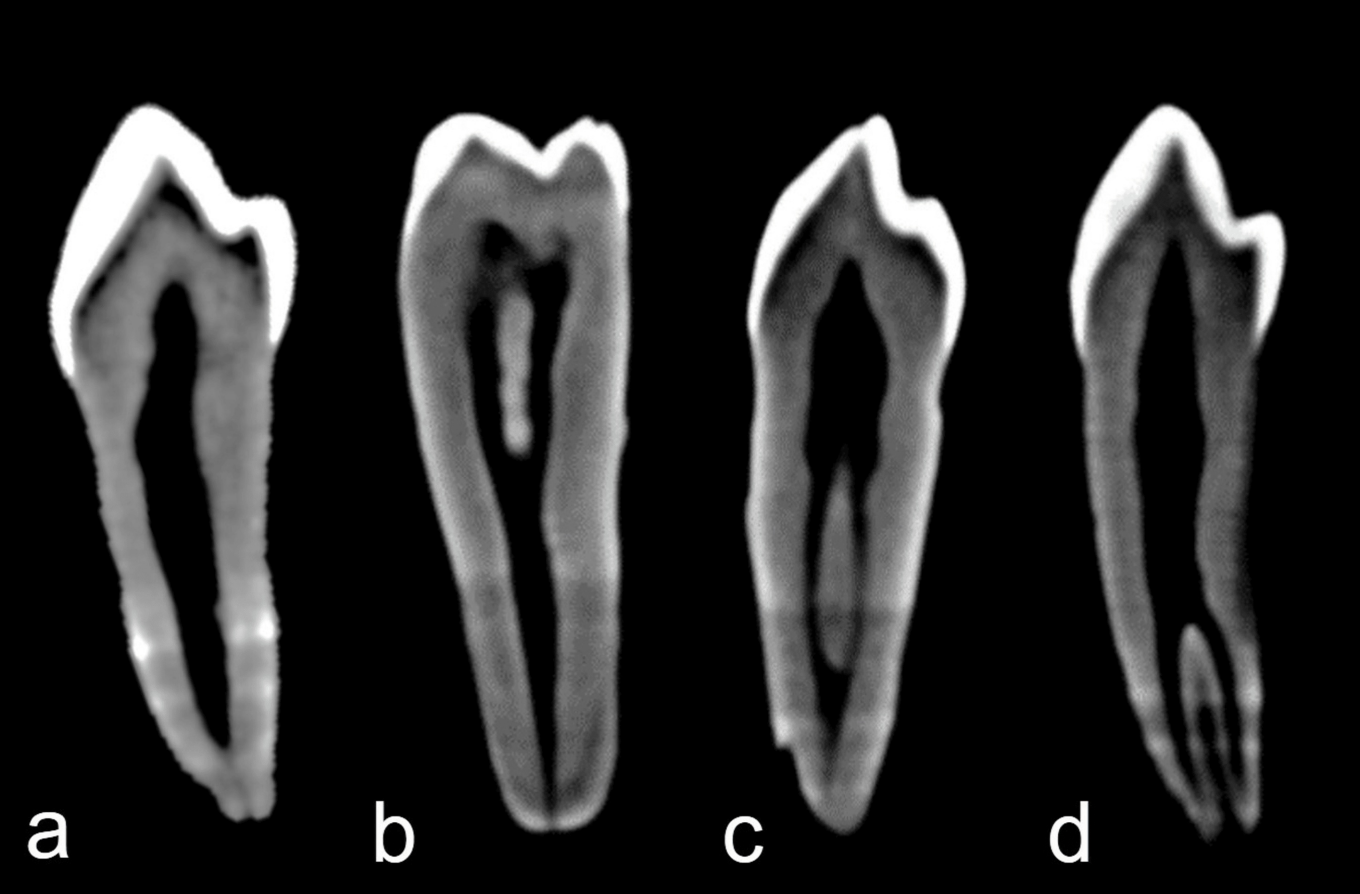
Cone beam computed tomographic images (sagittal section) of the types of canal configurations according to Vertucci’s classification found in the present study a) Type I, b) Type II, c) Type III, and, d) Type V
